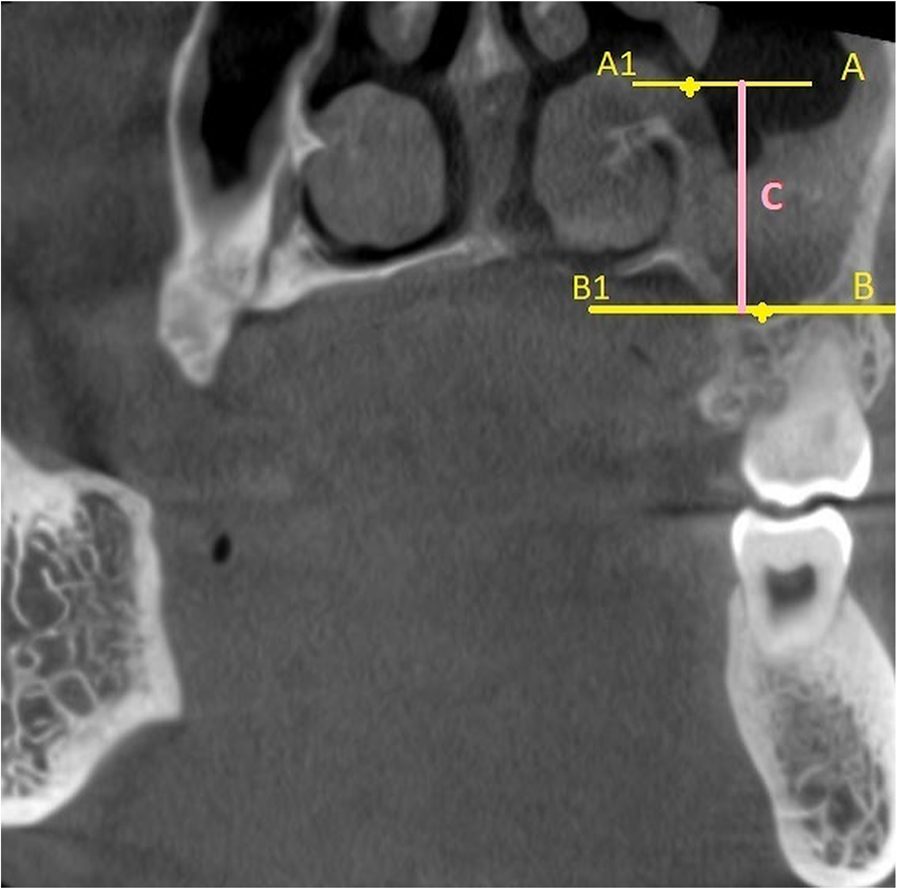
Coronal CBCT section showing method used to determine the location of AMO in vertical dimension (C). The distance C is obtained by measuring the distance between 2 horizontal lines A1-A (passing through the most inferior point of the AMO) and B1-B (passing through the most inferior point on the floor of the maxillary sinus)
